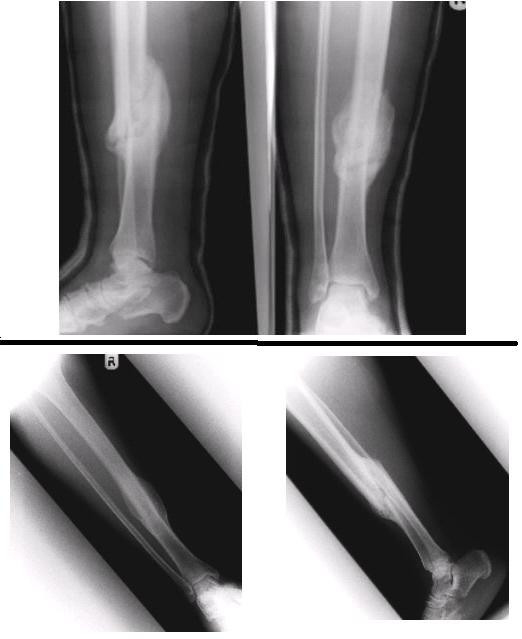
Radiograph 8 weeks and 2 years post injury.
